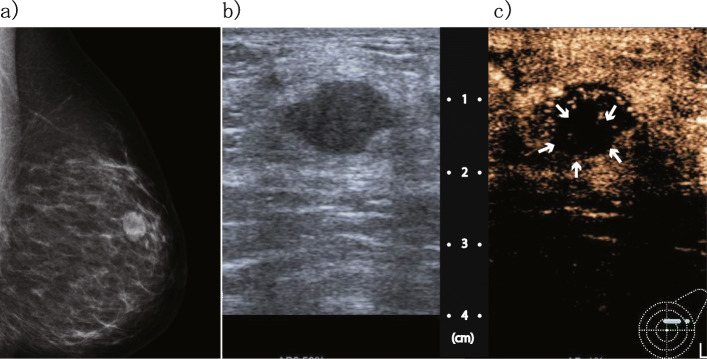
Imaging results. a Mammography; an equal density mass with calcification. b Ultrasound; a 1.4-cm microlobulated heterogeneous mass. c Contrast-enhanced ultrasound; arrows indicate contrast loss area
